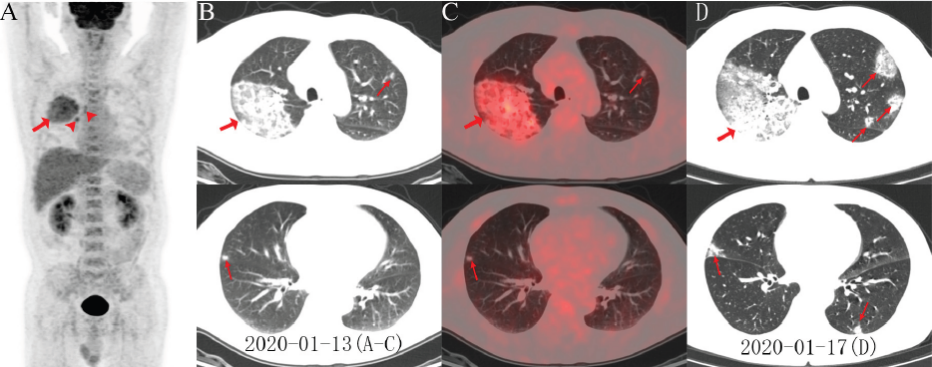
Fluorodeoxyglucose positron emission topography/computed tomography (FDG PET/CT) imaging findings in a patient with coronavirus disease. (A) The PET maximum intensity projection image shows an FDG-avid mass in the right lung with a maximum standardized uptake value of 4.9, as well as increased accumulation of FDG in the right hilar lymph nodes, in the right paratracheal stripe (arrowhead), and in the bone marrow. The axial images of the low-dose CT scan (B) and the PET/CT fusion (C) show ground-glass opacities in the right upper lobe with areas of focal consolidation (arrows) and focal opacities in the right middle and left upper lobes (arrows). Follow-up CT axial images obtained 4 days later (D) show lesion progression in the middle and bilateral upper lobes, with newly developed focal opacities in the left lower and upper lobes (arrows) [49].
